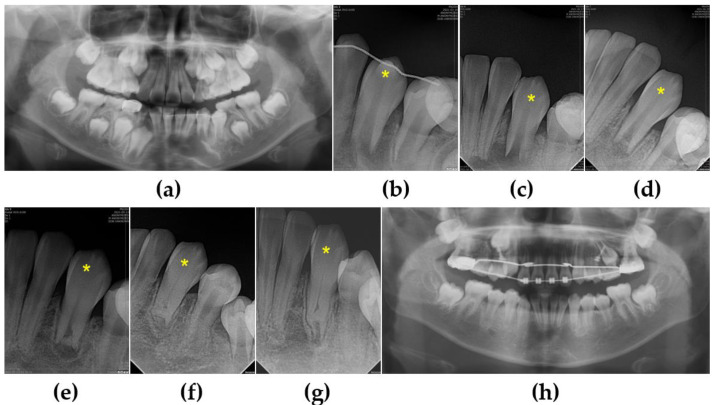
Radiographic findings. The transplanted tooth is marked with a yellow asterisk (*). Radiographs were taken on the day of autotransplantation (a,b), 1 month (c), 3 months (d), 6 months (e), 12 months (f), and 18 months (g,h) postoperatively.
